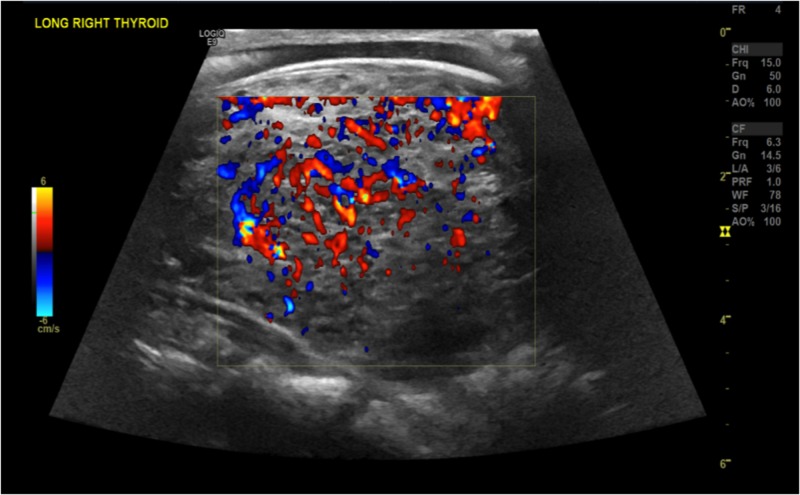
Doppler showing vascularity in the right thyroid lobe
